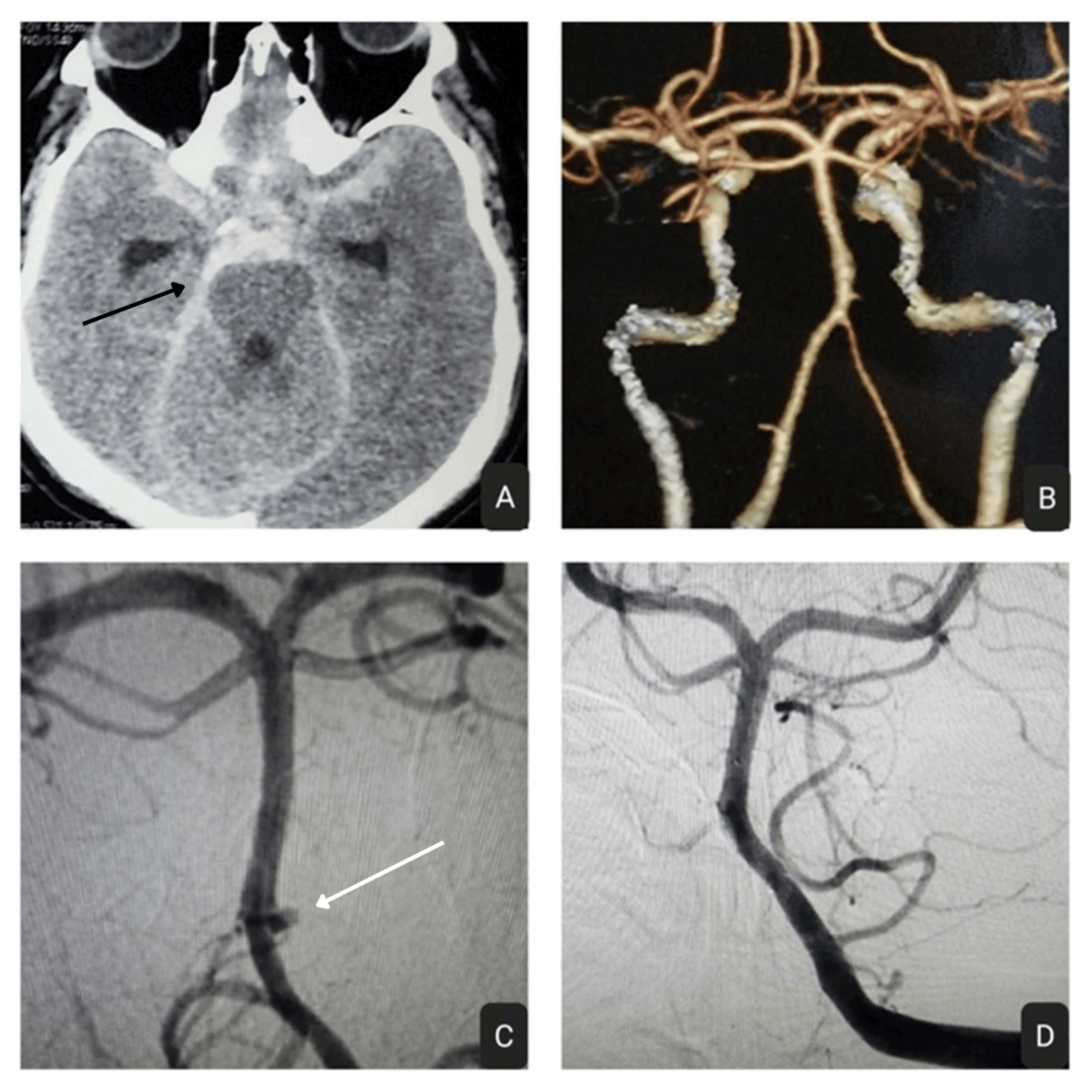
(A) CT Brain (plain) showing diffuse SAH (black arrow); (B) Proximal basila artery ruptured blister aneurysm seen on 3D CTA; (C) Diagnostic cerebral DSA; (D) Complete obliteration seen immediately after FDD placement SAH: sub-arachnoid haemorrhage; DSA: digital subtraction angiography; CT: computed tomography
